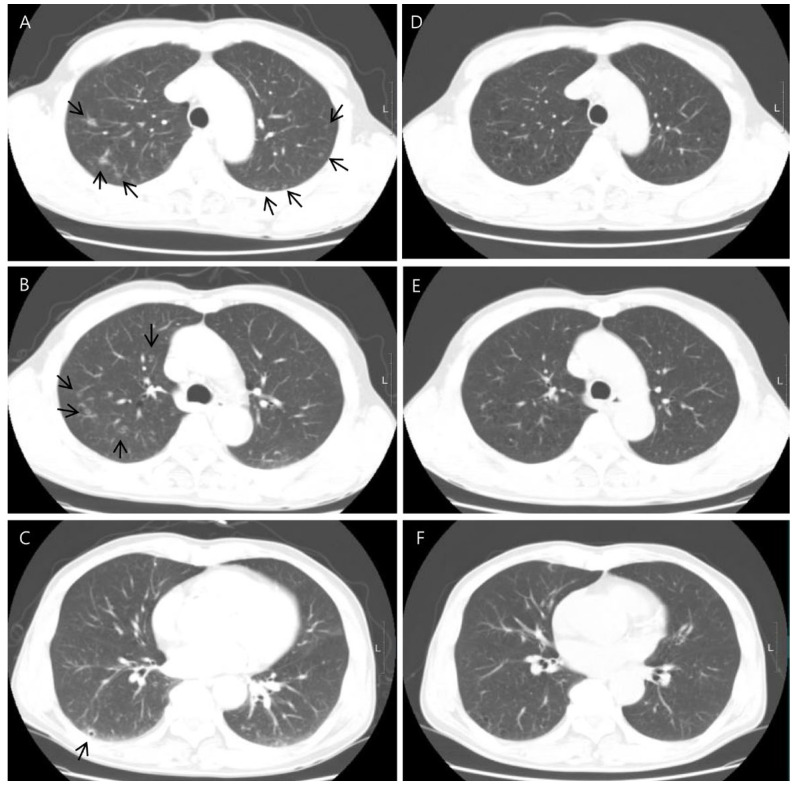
Axial chest CT showed multiple cavitary lung nodules in both lungs with upper lung zone predominance ((A–C), arrows). CT images obtained 5 months after showed the disappearance of the multiple cavitary nodules (D–F).
